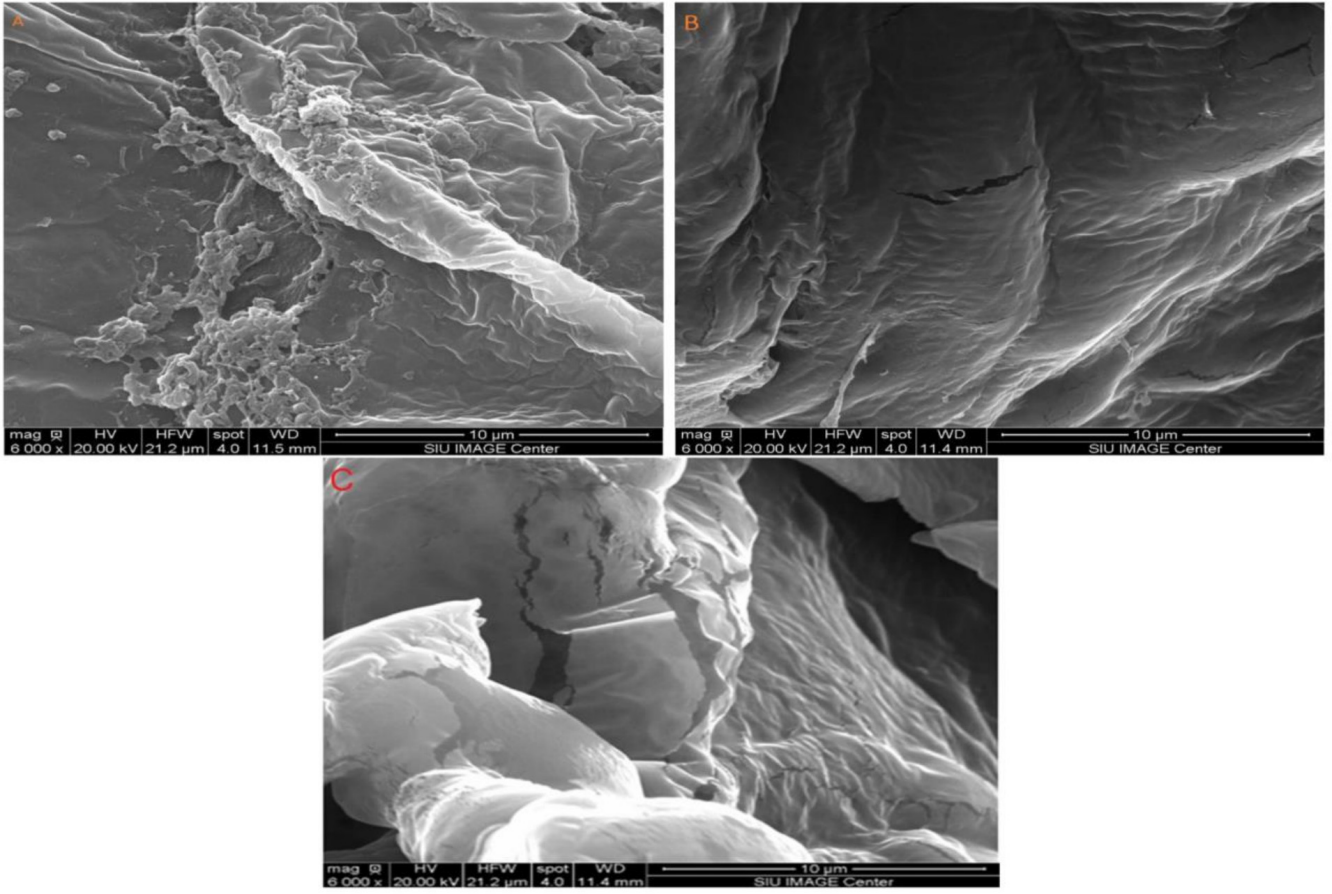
SEM images of pumpkin samples, Panel (A) a non-treated sample. Panel (B) a sample after solvent extraction. Panel (C) a sample after ultrasonic treatment.
